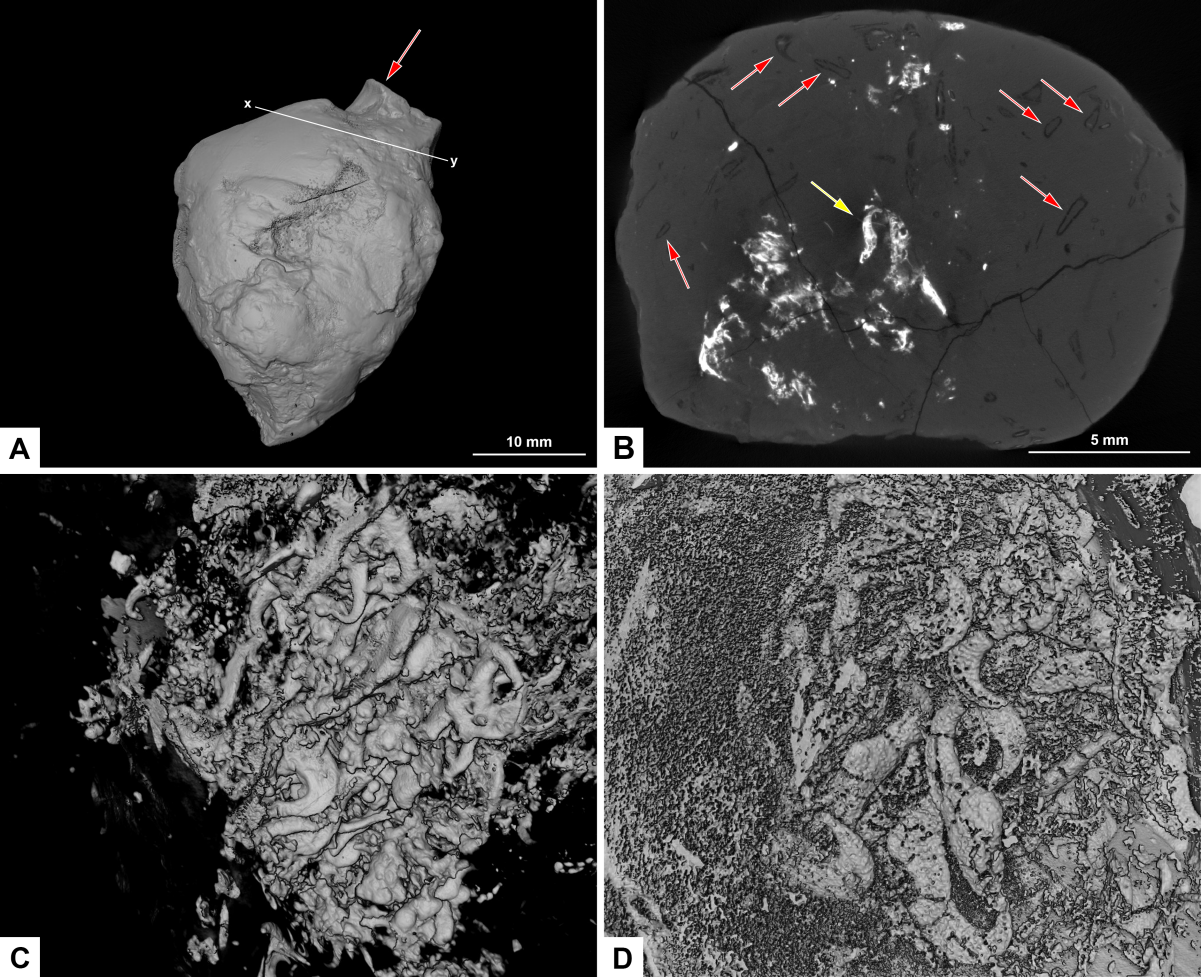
CT-scans of morphotype F, PMO 250.282. (A) External view of morphotype F with x-y being cross section seen in picture B. Red arrow pointing to bone stuck to the surface. (B) Orthoslice from x-y in picture A. Red arrows pointing to onychites with low density, yellow arrow pointing to onychites with high density. (C) Collection of onychites in high density. (D) Inverted CT-reconstruction showing a collection of onychites in low density.
